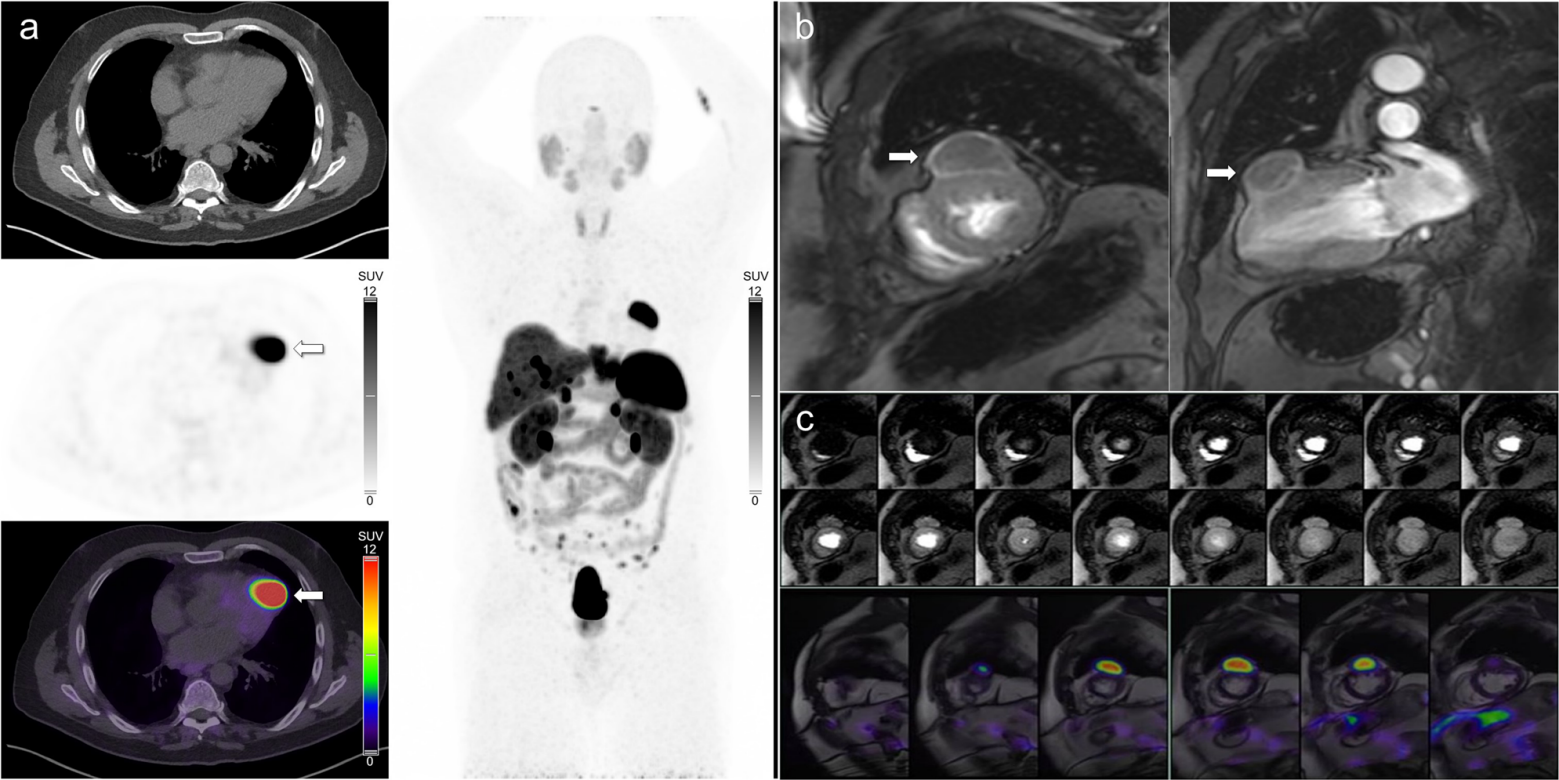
(A) [^68^Ga]Ga-DOTATATE PET/CT images in a patient with metastatic small bowel NEN demonstrating a large intensely avid metastasis in the mid anterior left ventricle (segment 7). Note the high target to background uptake and excellent lesion conspicuity. (B) Corresponding CMR images (T1-weighted delayed post-contrast short-axis and two-chamber views) demonstrate the same lesion. (C) Retrospectively fused short axis PET/MRI images of the same lesion
